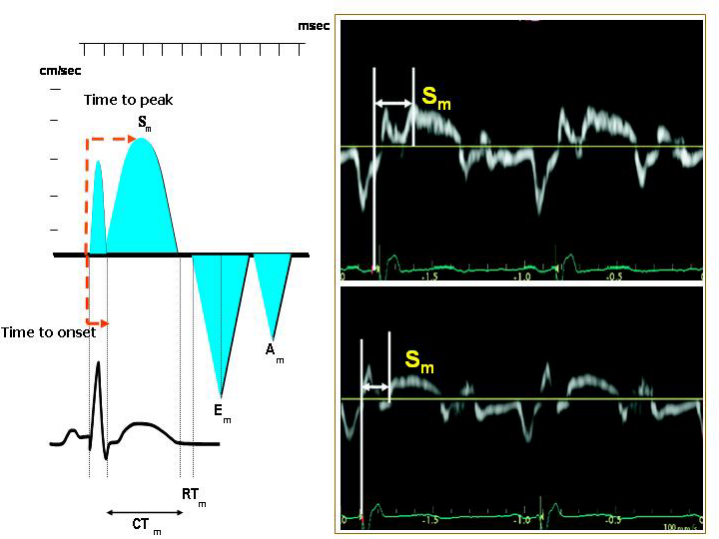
Methodology for measuring pulsed Tissue Doppler derived time to peak Sm and time to onset Sm (left panel). In the right panel measurements of time to peak Sm (upper panel) and of time to onset Sm (lower panel) are depicted. Am = Myocardial atrial velocity, CTm = Contraction time, Em = Myocardial early diastolic velocity, RTm = Myocardial relaxation time, Sm = Myocardial systolic velocity. Mod from Agler DA et al, *J Am Soc Echocardiogr *2007;20:76–90.
